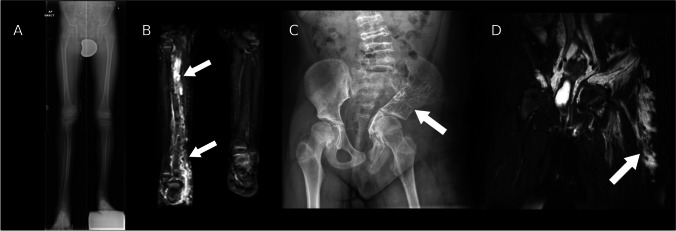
Stitched anteroposterior radiographs of the right femur (A) in a 52-year-old woman who presented to the emergency department with a spontaneous mid-diaphyseal femoral fracture on a background of dysplastic bone. An MR angiogram (B) revealed a complex arteriovenous malformation part of Klippel-Trenaunay-Weber syndrome
